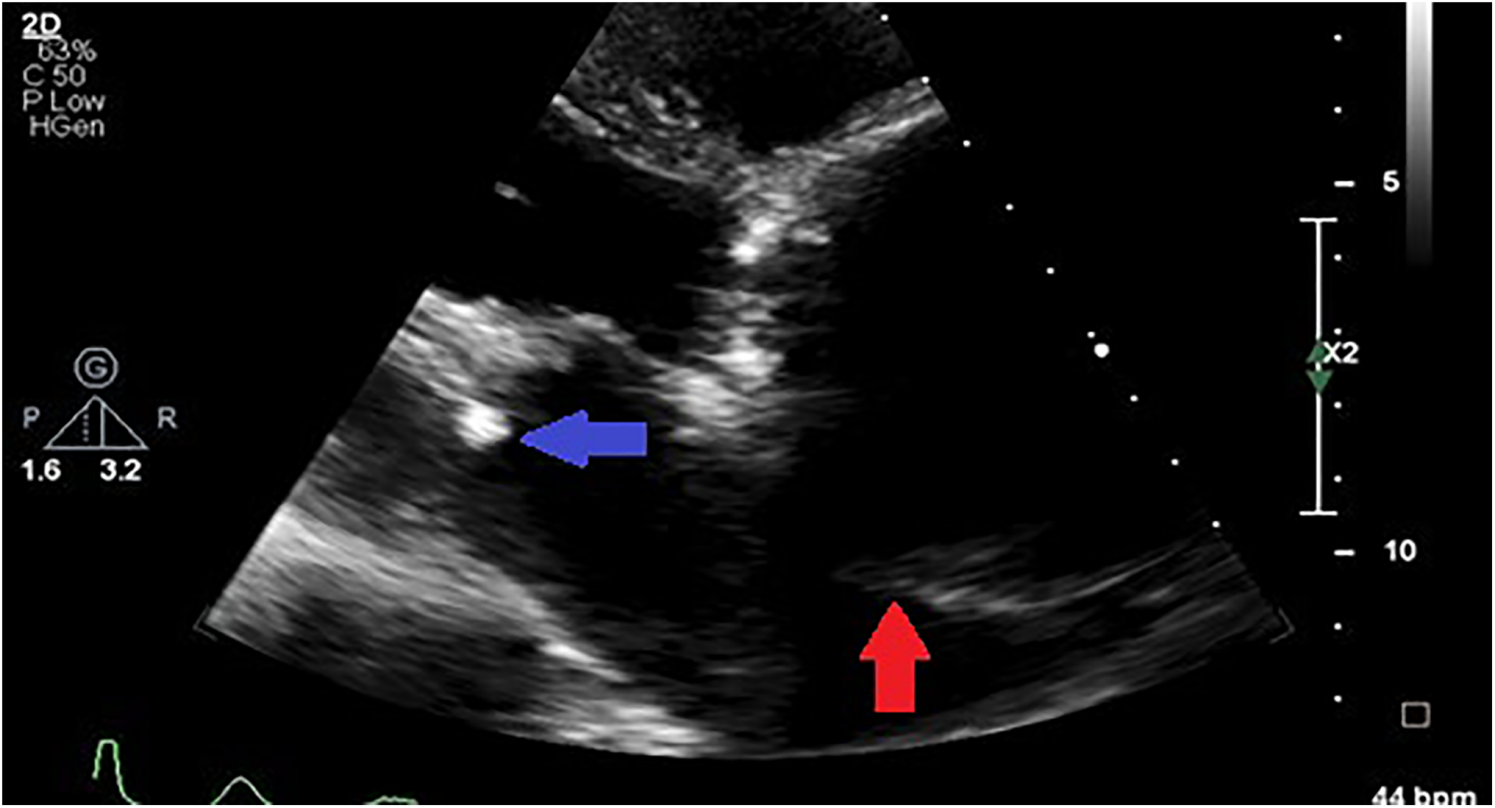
Parasternal off-axis view of the aorta in a hypertensive patient showing a markedly aneurysmal aorta compressing the left atrium (red arrow) and concurrent rheumatic mitral valve stenosis (blue arrow).
